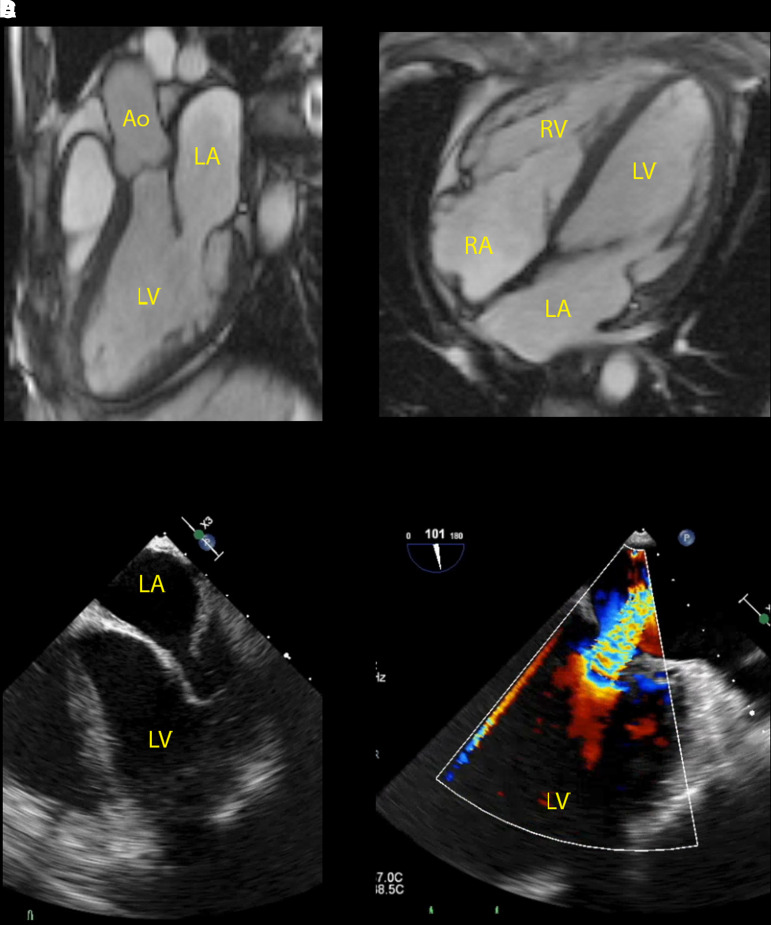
Imaging at first presentation showing severe mitral valve regurgitation with thickened mitral valve cusps and subvalvular apparatus on magnetic resonance imaging (*A* and *B*) and transoesophageal echocardiography (*C* and *D*). Ao, aorta; LA, left atrium; LV, left ventricle; RA, right atrium; RV, right ventricle.
